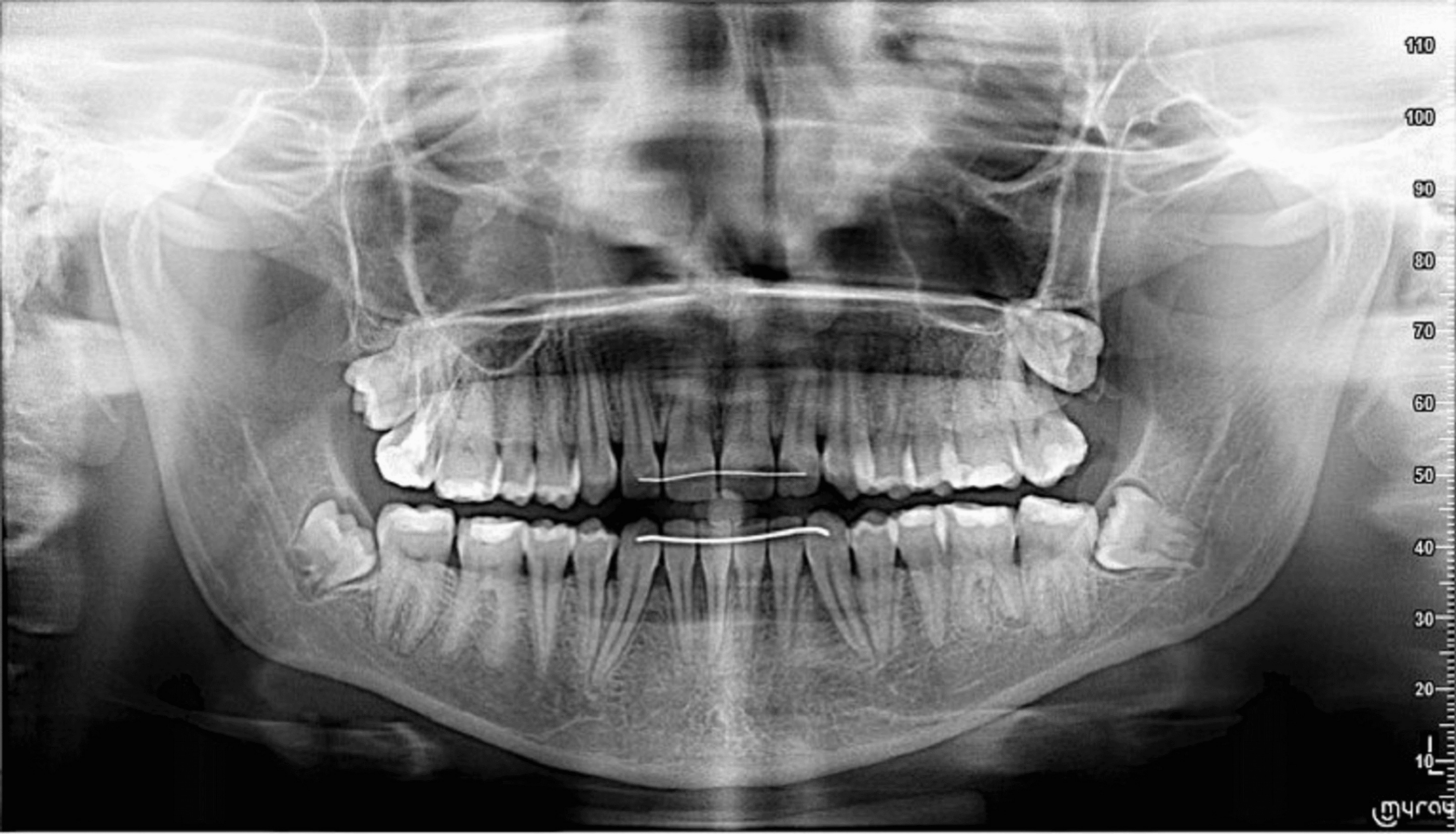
Panoramic radiograph obtained after treatment. Panoramic radiograph demonstrating overall alignment and root parallelism.
